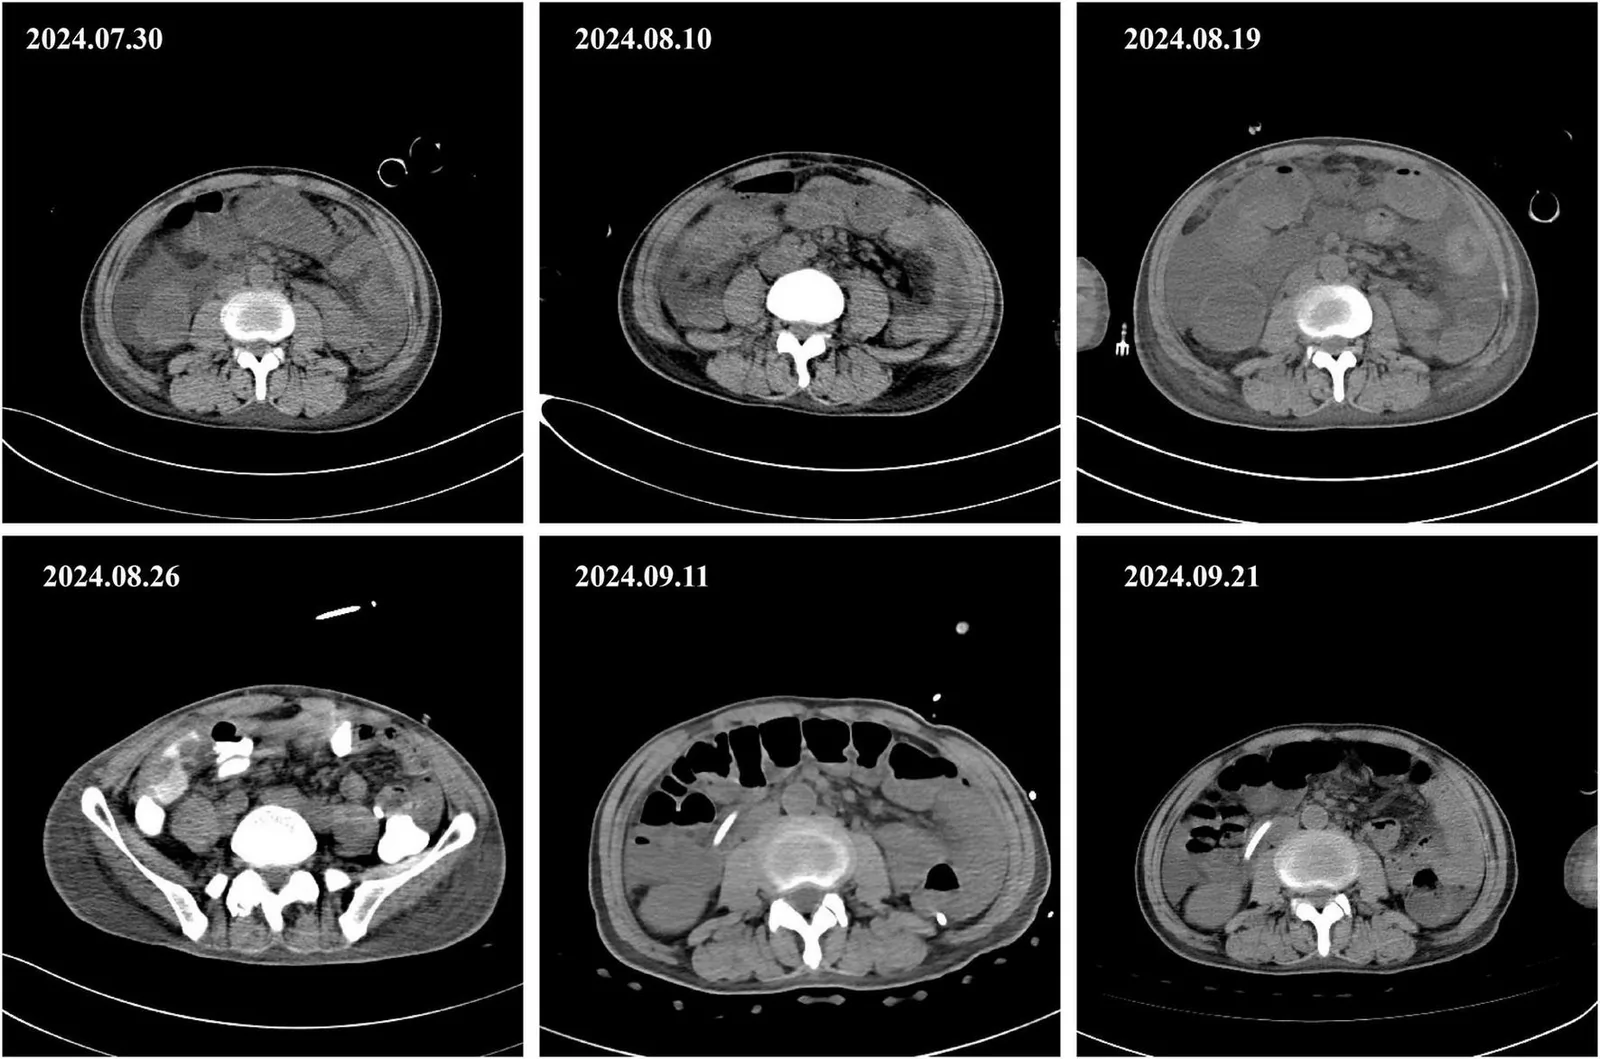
Serial abdominal CT images show evolution from massive ascites to a right lower quadrant cystic lesion containing a small amount of gas, with complete resolution of both by day 65, paralleling the decline of ascitic fluid amylase and fungal clearance.
